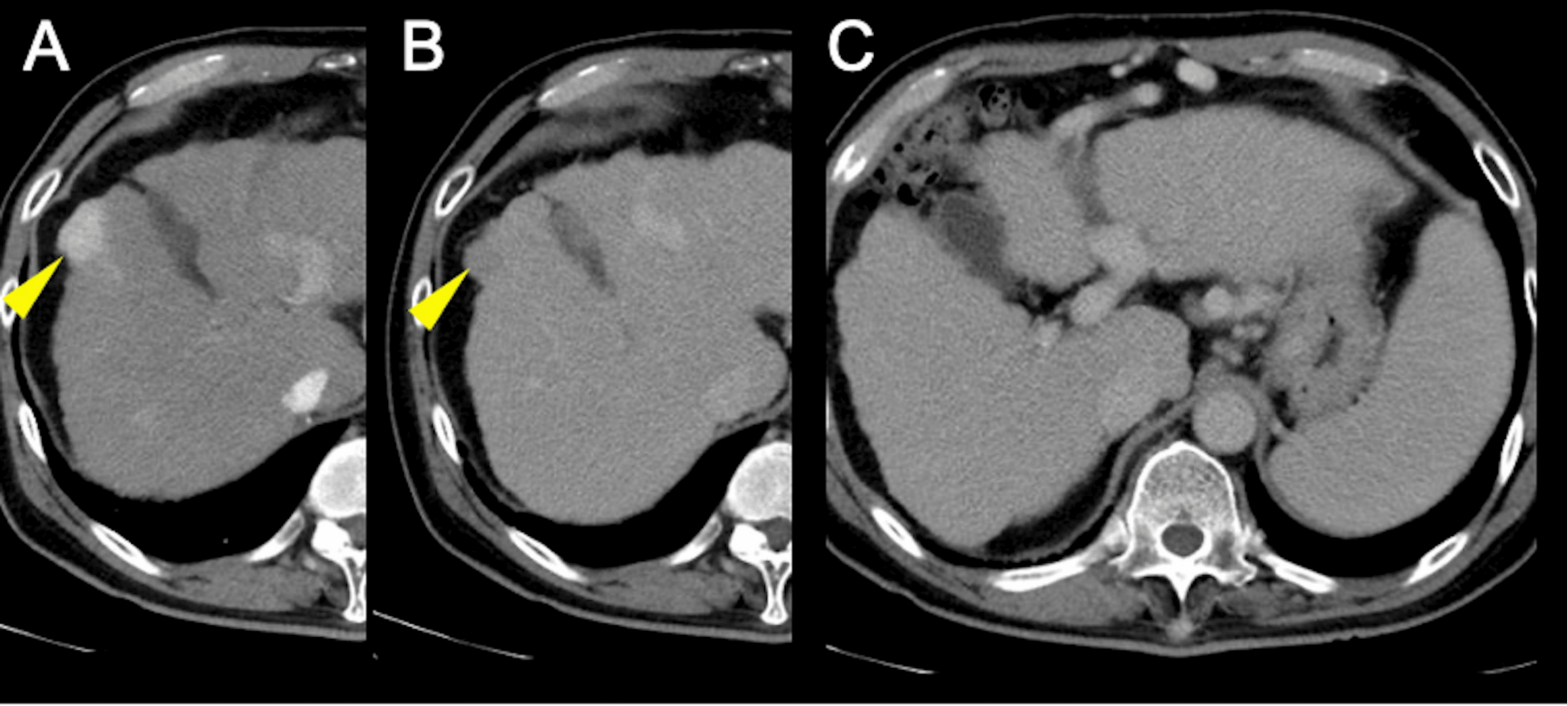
Radiological findings of hepatocellular carcinoma with splenomegaly (A, B) On contrast-enhanced axial CT, a 2-cm lesion in hepatic segment five shows early arterial enhancement and subsequent washout (yellow arrow), consistent with HCC. (C) The spleen is massively enlarged, and multiple collateral vessels are observed around it, indicating advanced portal hypertension. The liver measured approximately 18 cm in craniocaudal dimension at the mid-clavicular line. No free intraperitoneal fluid was detected.
